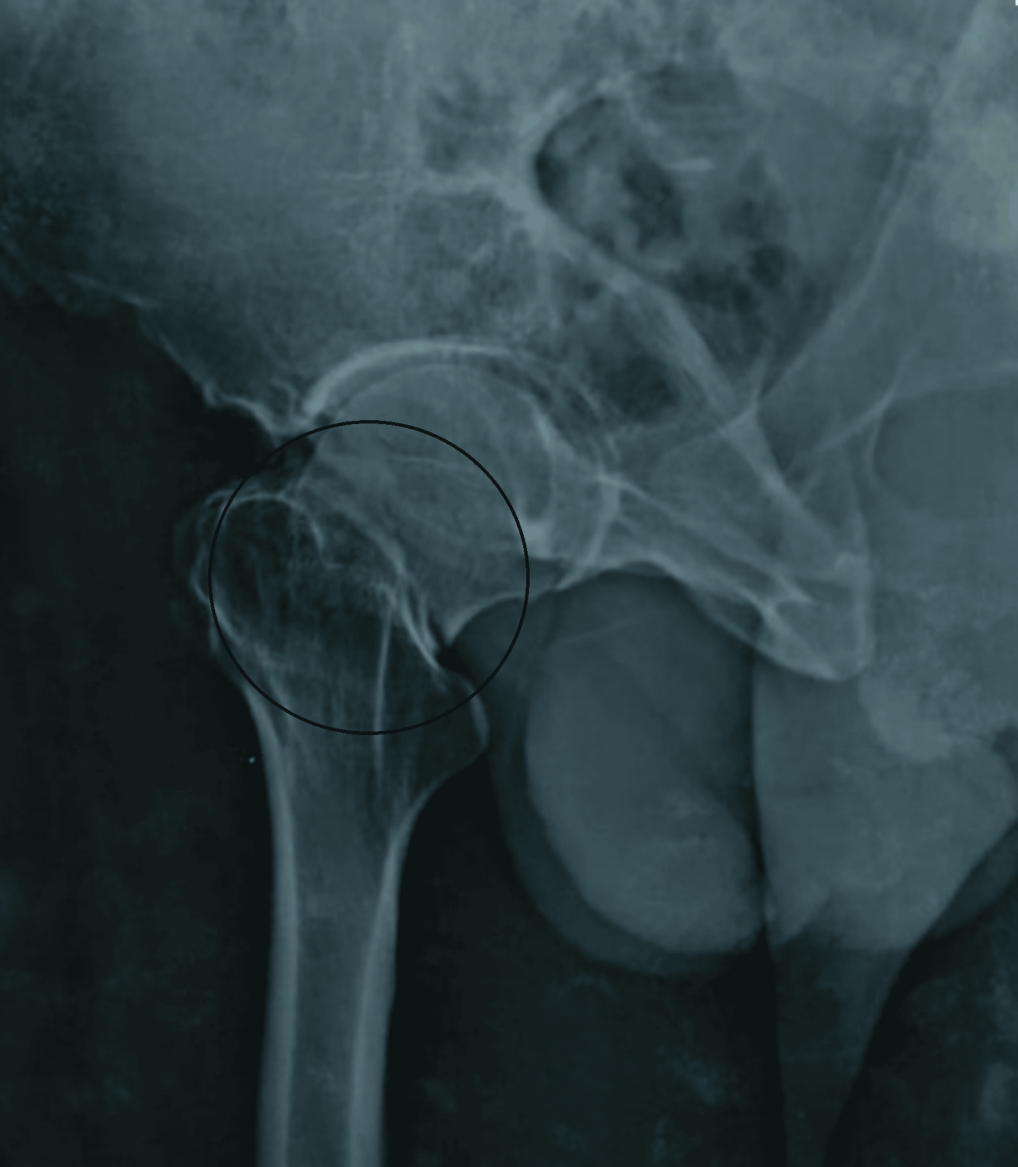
X-ray of the right hip (cross-table lateral) showing right-sided femoral neck fracture (Garden Type IV and Pauwels Type III)
